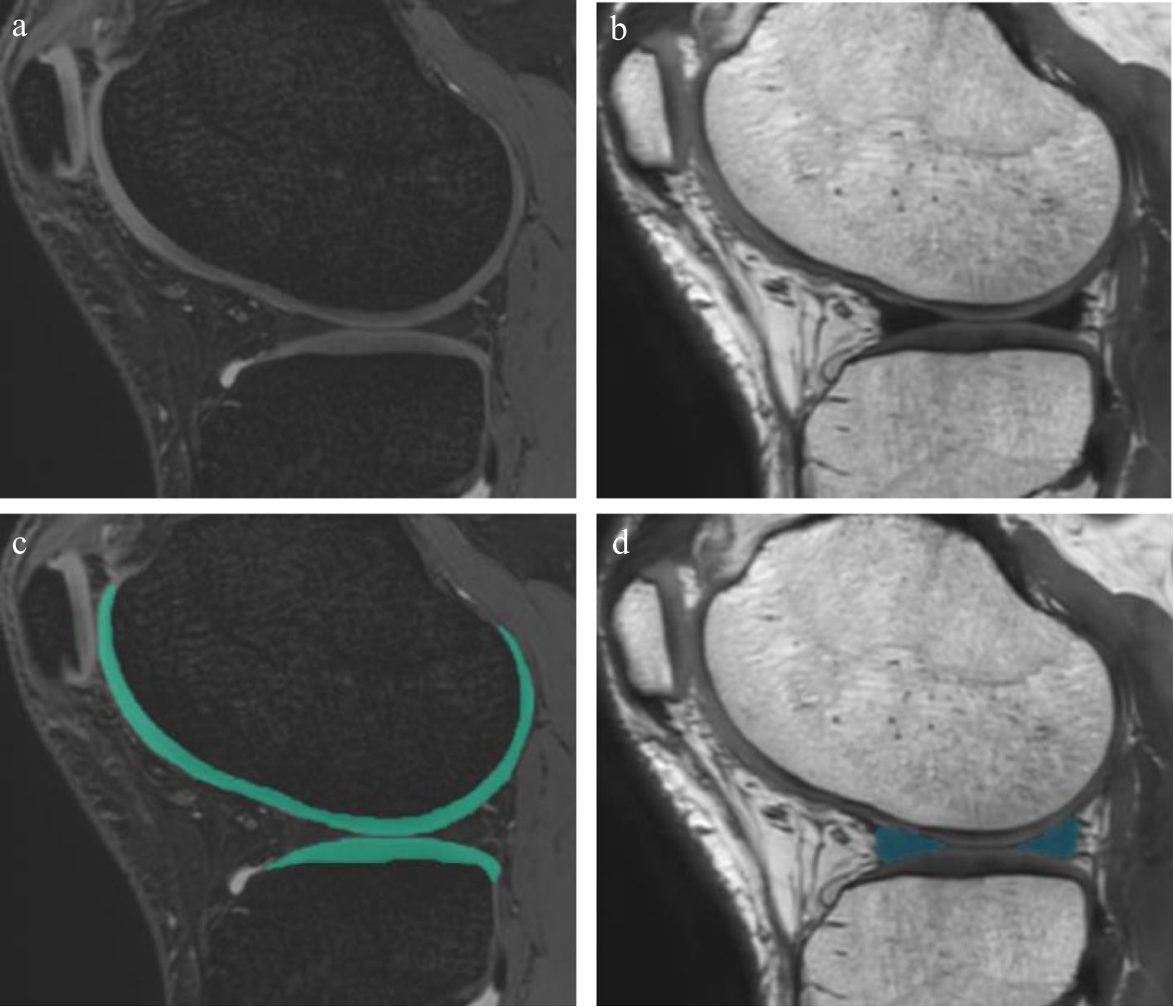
2D MRI of the knee for 3D analyses. a Fat-suppressed spoiled gradient echo (SPGR) image. b Proton density weighted imaging 3D fast spin echo/ turbo spin echo (PDWI 3D FSE/TSE) image. c Femoral and tibial cartilage automatically extracted from SPGR image. The extracted cartilage is shown in green. d The meniscus automatically extracted from PDWI 3D FSE/TSE image. The extracted meniscus is shown in blue
